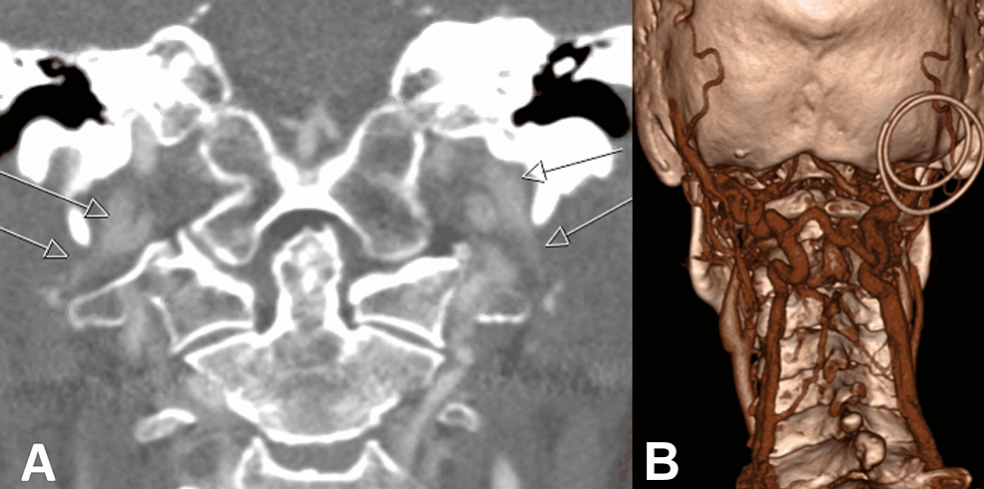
Coronal view of CT angiogram. The scans demonstrating bilateral narrowing of IJVs at the junction between the styloid processes and C1 lateral mass (arrows) (A). Large, tortuous, engorged anastomotic venous plexus crossing the midline, with collaterals to the posterior jugular vein and bilateral vertebral veins (B). IJV: internal jugular vein
